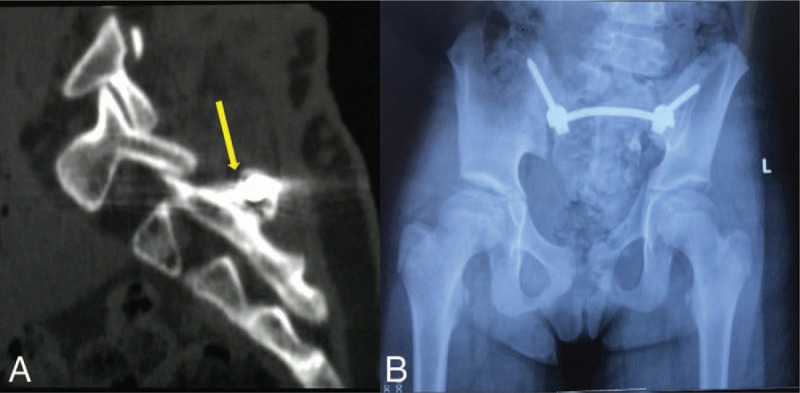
(A) Postoperative CT confirmed that the connecting rod passed through the spinous process completely. (B) X-ray suggested corrected pelvis floating in the standing position. CT = computed tomography.
